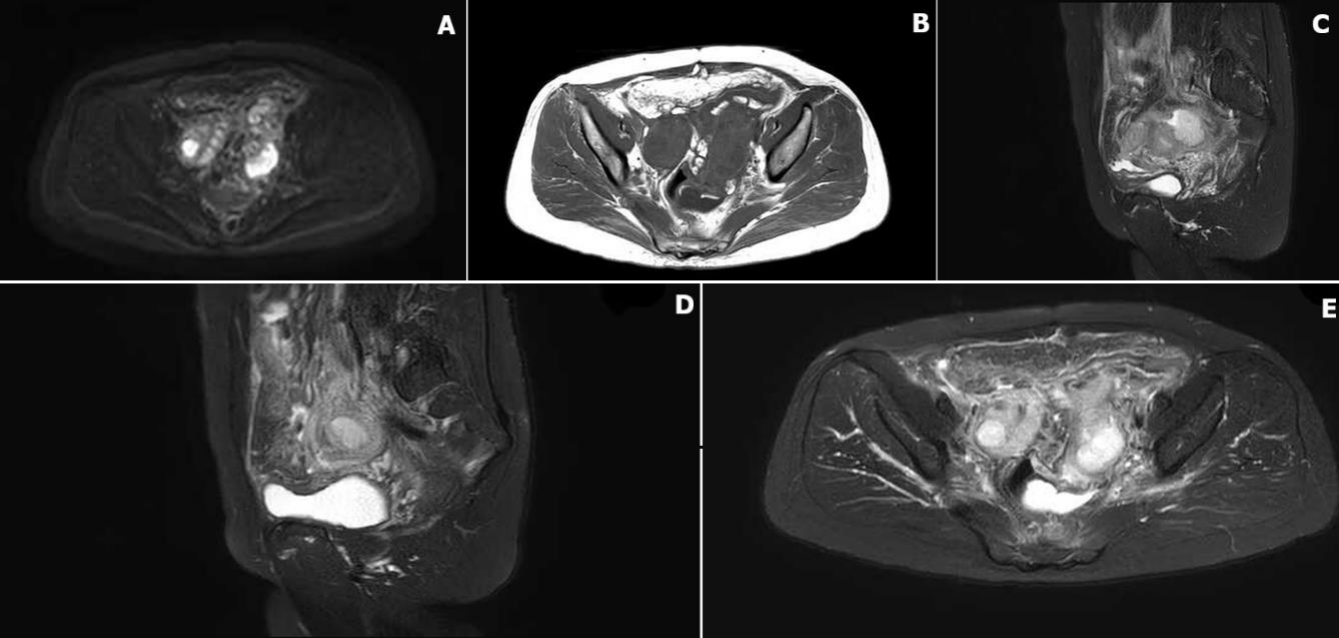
pelvic MRI: A) DWI: restricted diffusion, purulent cysts; B) axial T1WI: isointense to myometrium; C,D) sagittal T2WI: hyperintense masses, cystic foci; E) axial T2WI: complex cystic-solid lesions, stranding
